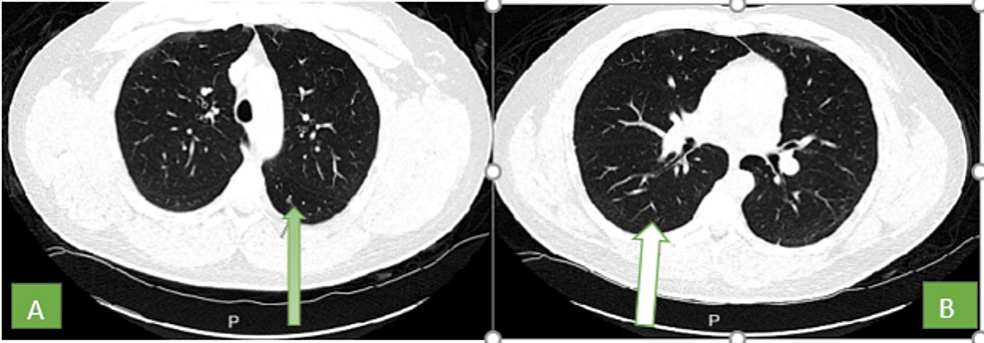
CT of the chest without IV contrast. A: Linear opacity along the dependent region of the left lower lobe; finding represents scarring, and ground glass nodule within the superior segment of the left lower lobe measuring approximately 4 mm (thick green arrow). B: There is a subpleural nodule along the posterior aspect of the right lower lobe superior segment measuring approximately 4.1 mm. There are ground glass opacities at the periphery of bilateral upper lobes anteriorly, probably interstitial lung disease.
